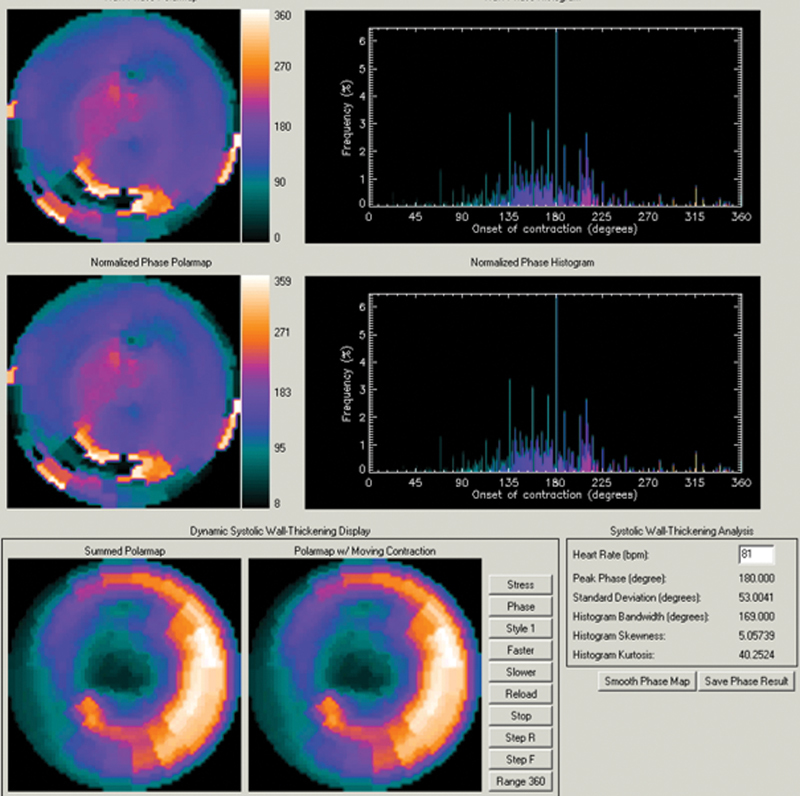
Assessment of LVMD by GSPECT MPI.
